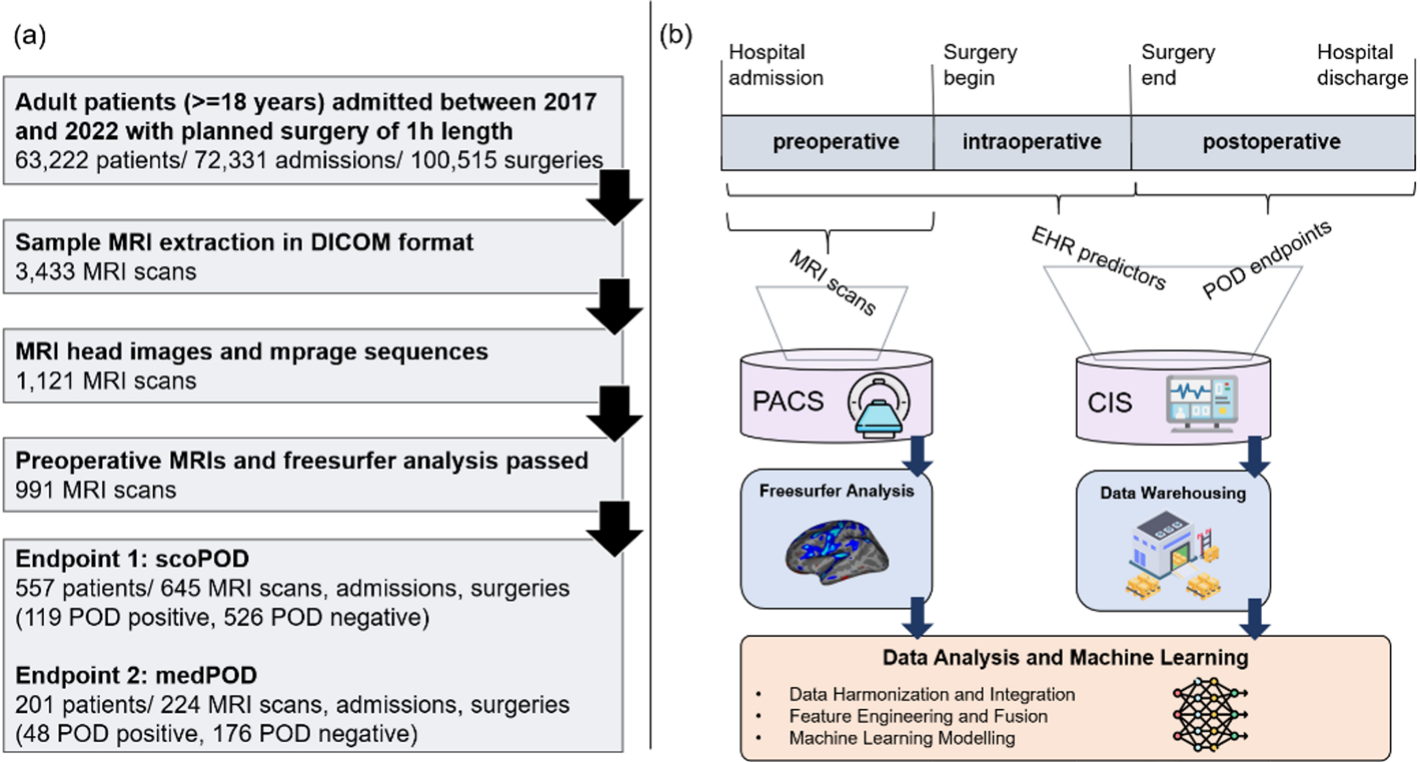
Inclusion criteria for endpoint definitions (a), methodology and data flow (b). We included data of all adult patients between 2017 and 2022 with estimated surgery length of at least one hour. MRI headers from DICOM metadata were filtered for head-scans, MPRAGE sequences, and scanning time. Preoperative MPRAGE sequences that did not pass automated segmentation (e.g. extreme movement) were excluded (shown in a). We divided the hospital stay into pre-, intra-, and postoperative time phases. MRI scans were extracted from the picture archiving and communication system (PACS), EHR data were extracted from the clinical information system (CIS) for integrated data analysis and the application of machine learning (shown in b).
